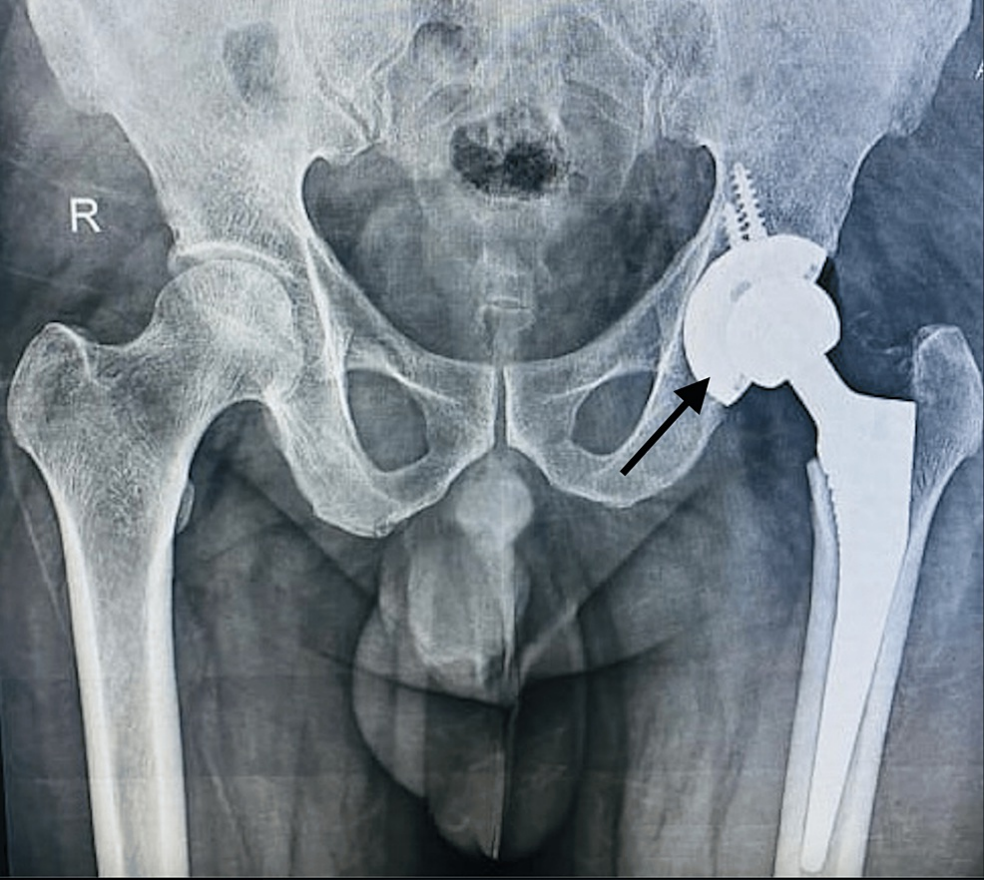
X-ray of the pelvis with both hips with total hip arthroplasty on the left side
